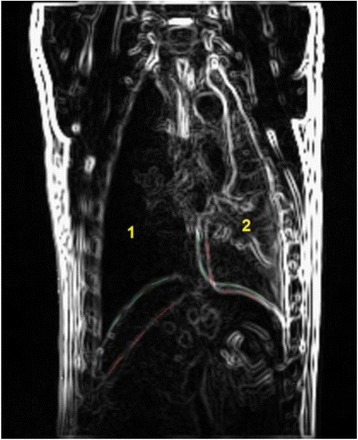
Merged MR images at endex-inspration after edge detection, diaphragm position in ex- (green) and inspiration (red), ventilated (1) and flooded (2) lung
